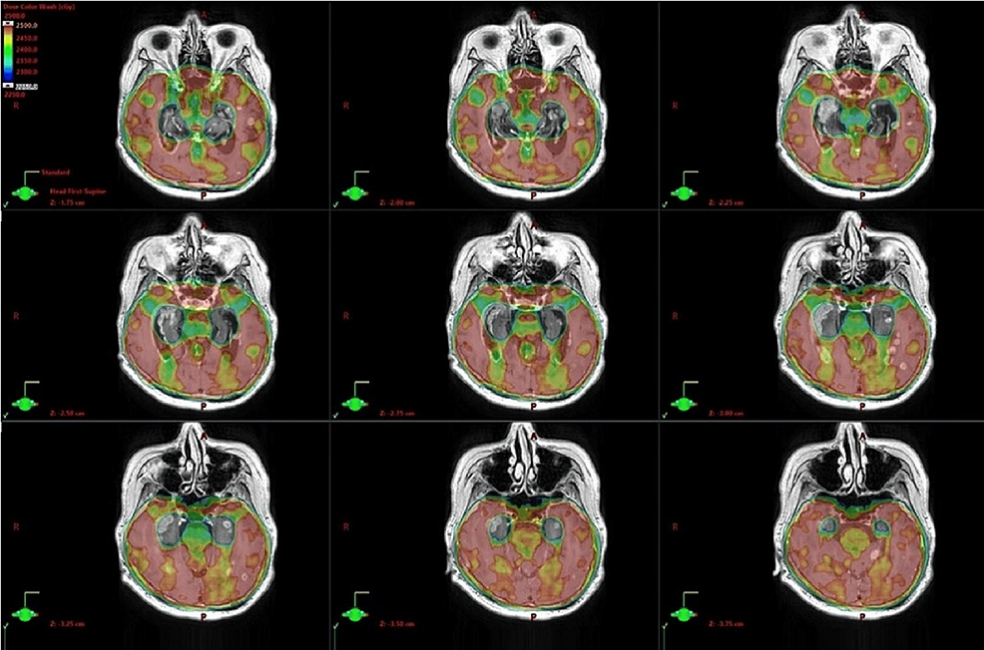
Registration image: MRI of meningeal carcinomatosis and dose distribution map for hippocampal avoidance prophylactic cranial irradiation. The images display multi-slice axial registration, which fuses the magnetic resonance images of meningeal carcinomatosis with the dose distribution map of hippocampal avoidance prophylactic cranial irradiation. The color wash dose range is 22.5Gy to 25Gy. These registration images, created using the Eclipse treatment planning system (version 16.0, Varian Medical Systems), clearly show that the area avoiding the hippocampus overlaps with the main lesion of meningeal carcinomatosis.
